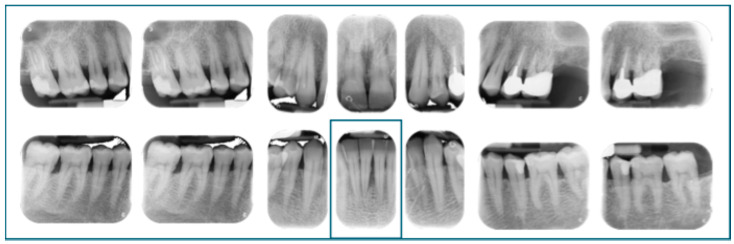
Full-mouth radiographs reveal localized Stage III periodontitis, evidenced by bone loss extending beyond the middle third of the root at tooth 4.1. The narrative summary of this case could also reflects that the remaining dentition is affected by generalized Stage II periodontitis.
